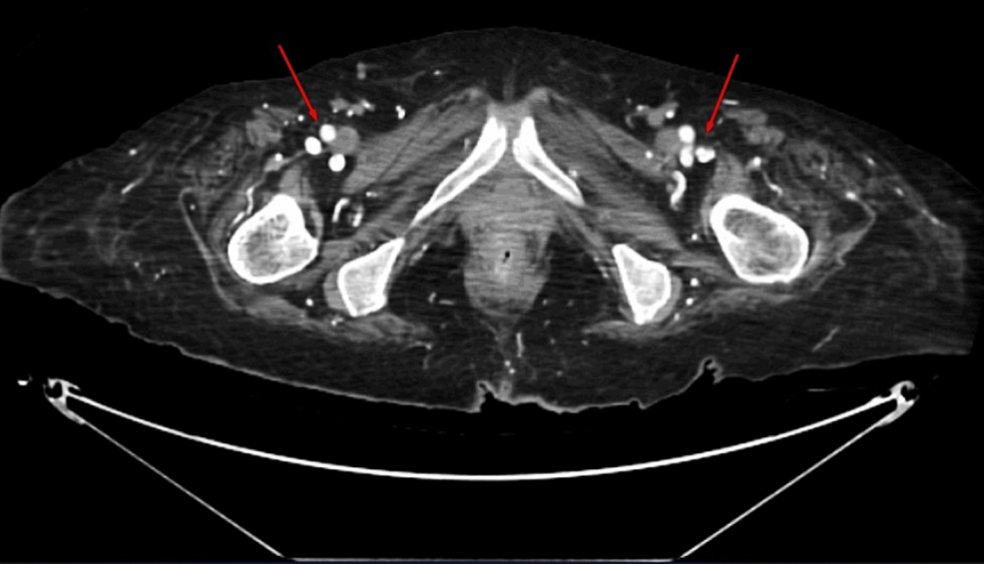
Computed tomography of the thigh with contrast showed extensive arterial calcifications.
